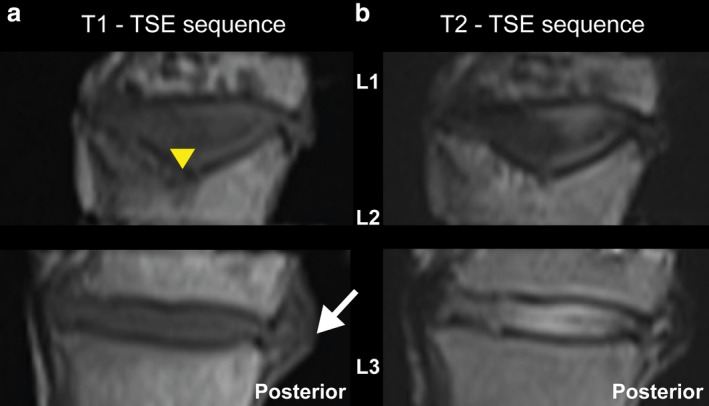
MRI scan (Turbo Spin Echo: TSE): (a) sagittal T1 and (b) T2. The intervertebral disc between L1 and L2 is expanded into the collapsed superior L2 (yellow triangle). A prolapse of the intervertebral disc between L2 and L3 can be detected in the T1‐weighted MRI scan (arrow). In the T2‐weighted MRI image, the intervertebral disc between L2 and L3 is characterized by an increased signal intensity.
